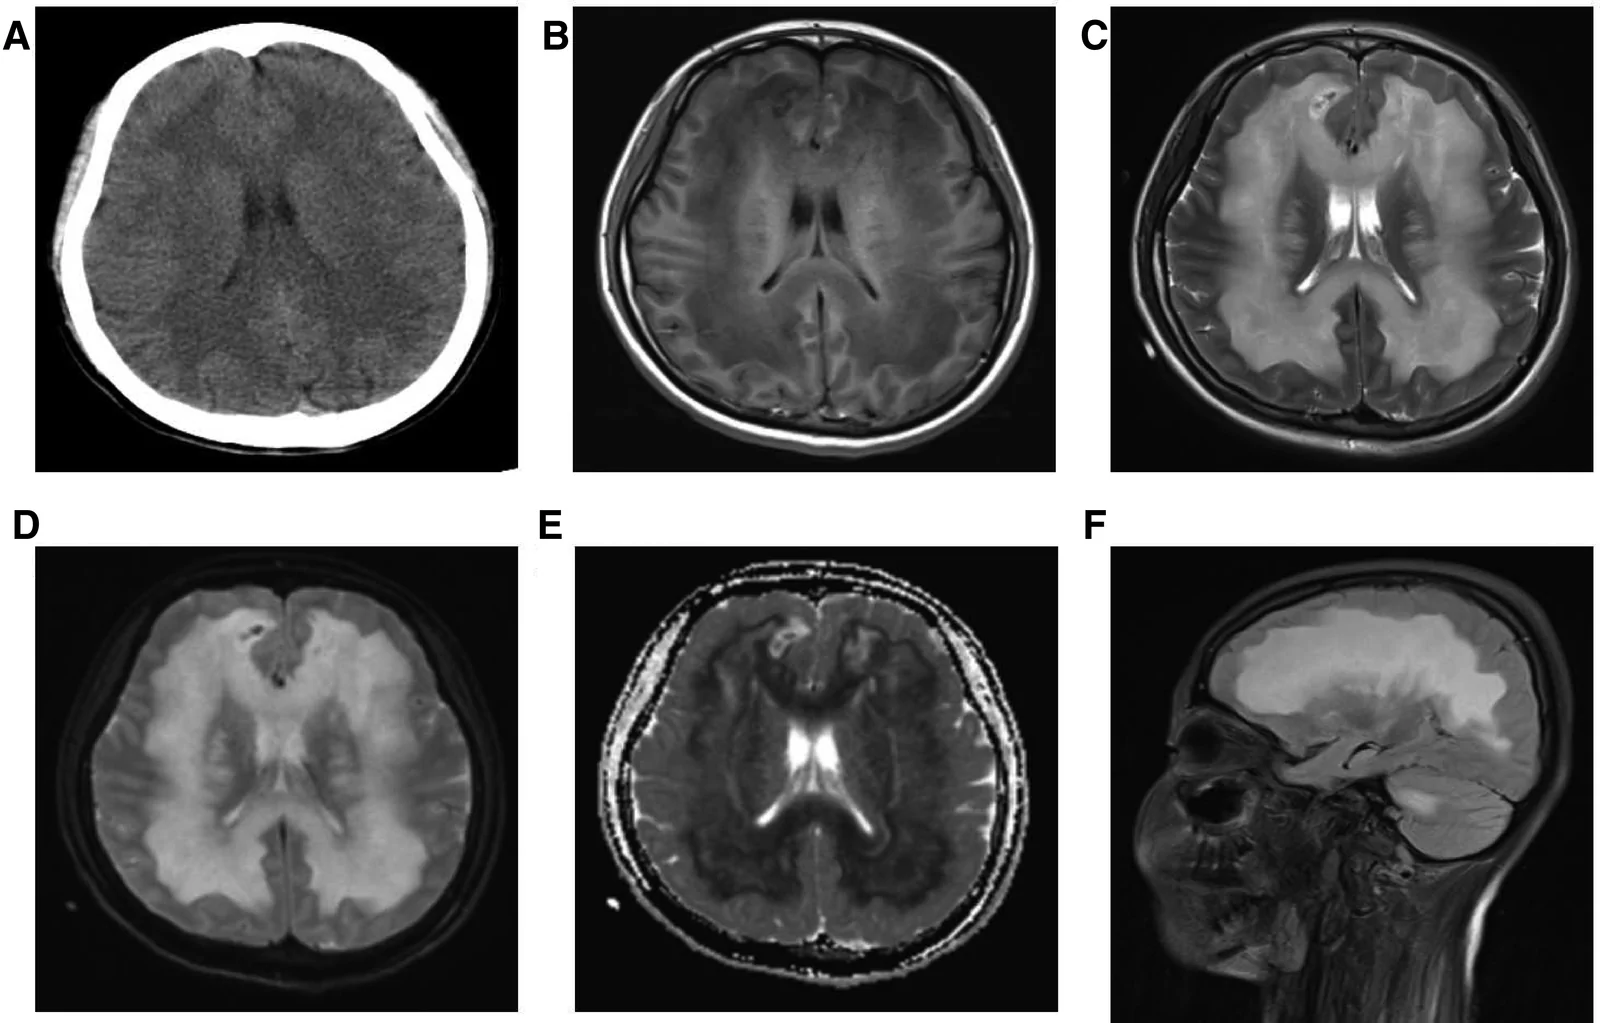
Neuroimaging findings in Case 1. (A) Initial non-contrast computed tomography (CT) showed no acute intracranial abnormalities. (B) Coronal T1-weighted image (T1WI), (C) coronal T2-weighted image (T2WI), (D) diffusion-weighted imaging (DWI), and (E) apparent diffusion coefficient (ADC) map demonstrated bilateral symmetrical lesions involving the thalami, lenticular nuclei, corpus callosum, and cerebral white matter. (F) Sagittal T2WI demonstrated additional involvement of the cerebellum and cerebral white matter.
